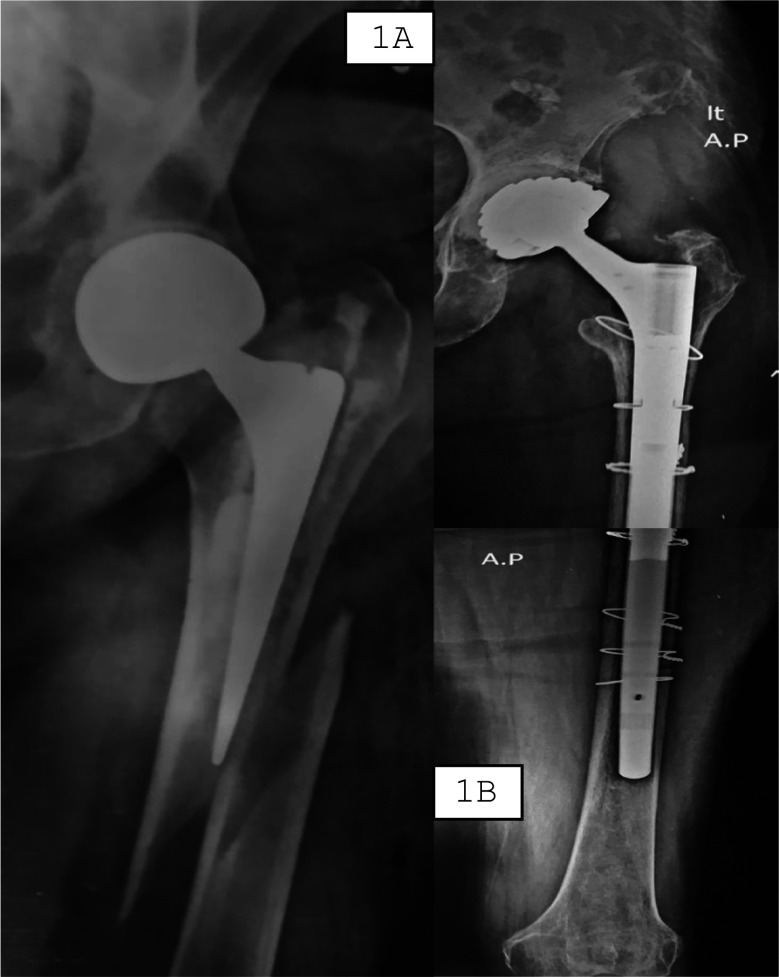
A 58-year-old male patient who underwent revision total hip arthroplasty using a modular cementless long stem (Revitan^™^) for a periprosthetic femoral fracture. A AP radiograph of the left hip and femur showing the fracture pattern prior to revision. B Final follow-up AP radiograph demonstrating stable fixation, satisfactory alignment, and successful integration of the modular long stem
